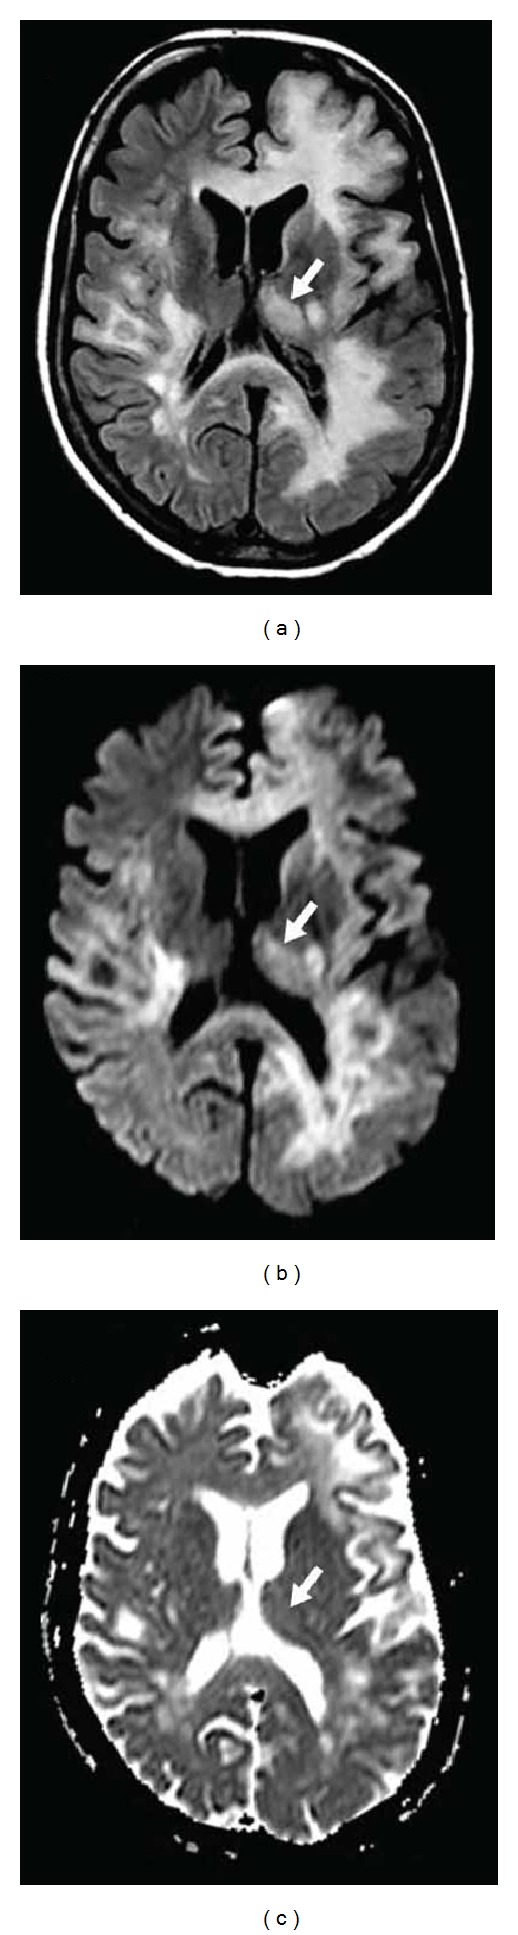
Diffuse white matter and associated left frontal cortical and left thalamic (arrows) involvement seen as hyperintensity on FLAIR (a), DWI (b), and ADC map (c) in a patient with natalizumab-related (for multiple sclerosis) progressive multifocal leukoencephalopathy.
